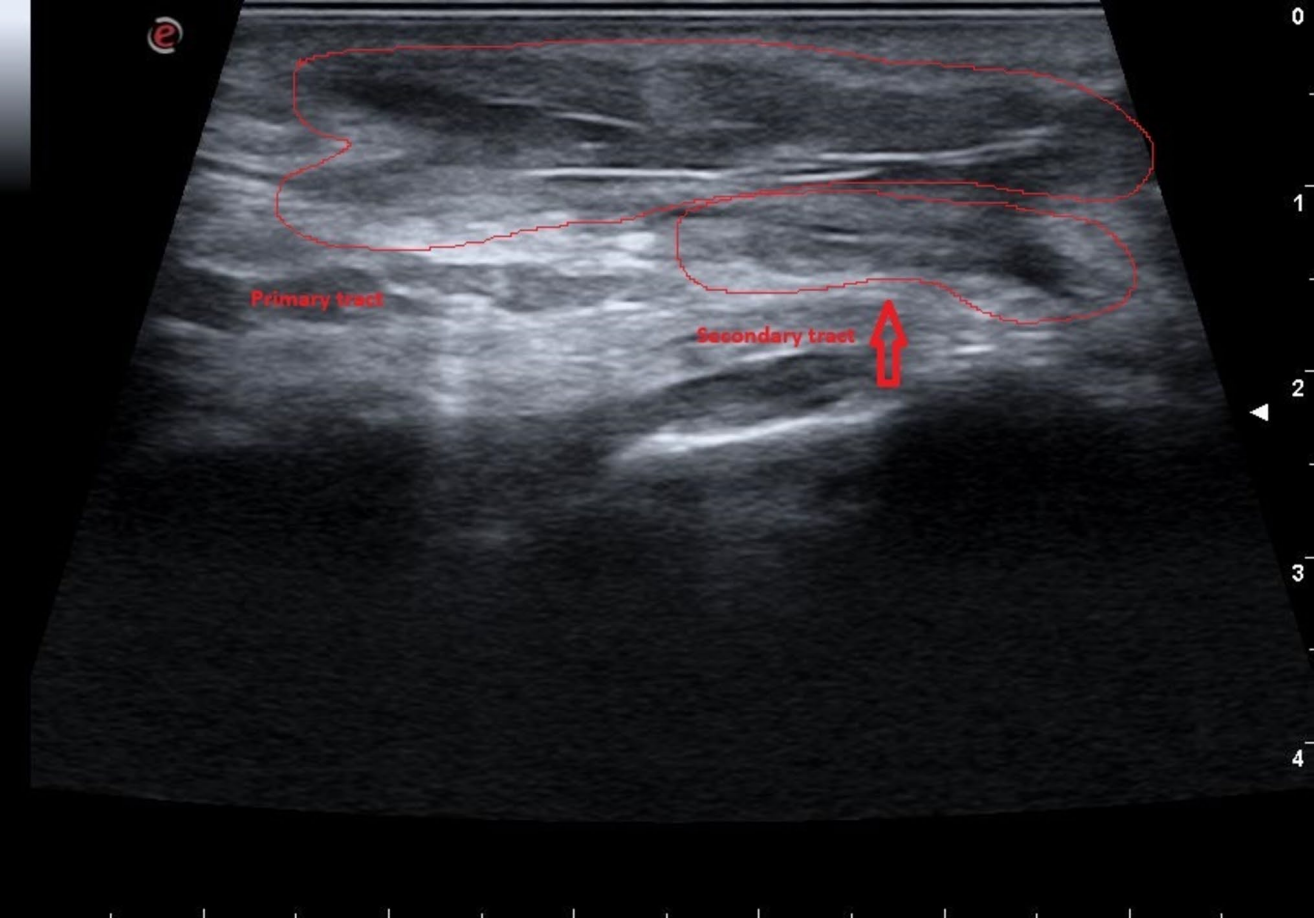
Ultrasonographic identification of both primary and secondary (deeper and potentially unrecognizable without the use of IUS) fistulous tracts
